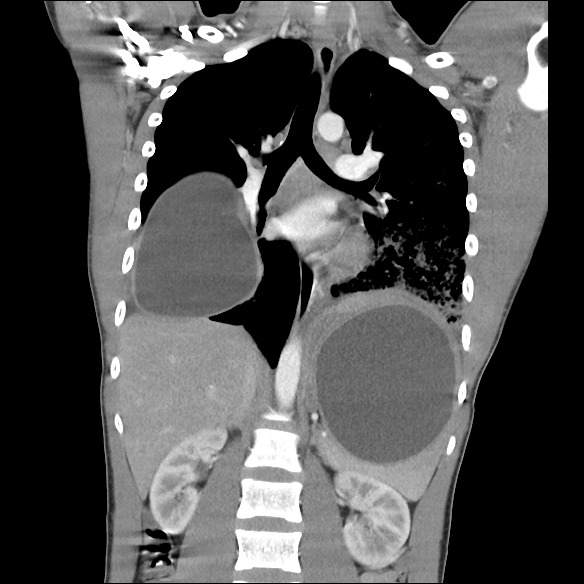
Computed tomography chest scan (axial view).
